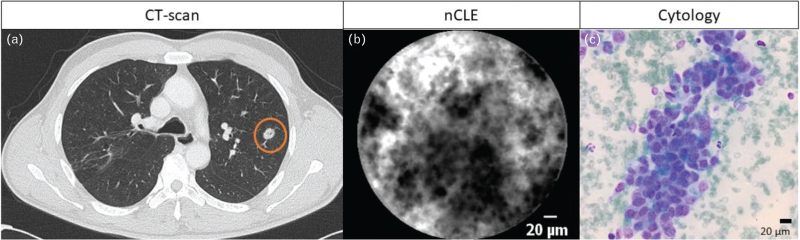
Bronchoscopic nCLE-imaging of a peripheral lung tumour. (a) Chest CT-scan with a lung tumour in the left lower lobe (circle). (b) nCLE-imaging of the lung lesion shows clusters of enlarged pleomorphic tumour cells. (c) Corresponding cytology representing a pulmonary metastasis of a urothelial carcinoma.
